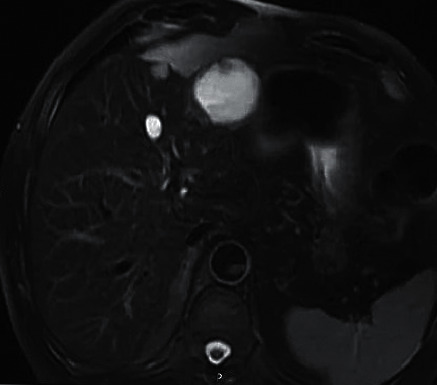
An MRI image of a male HCH patient (aged 61 years old).
